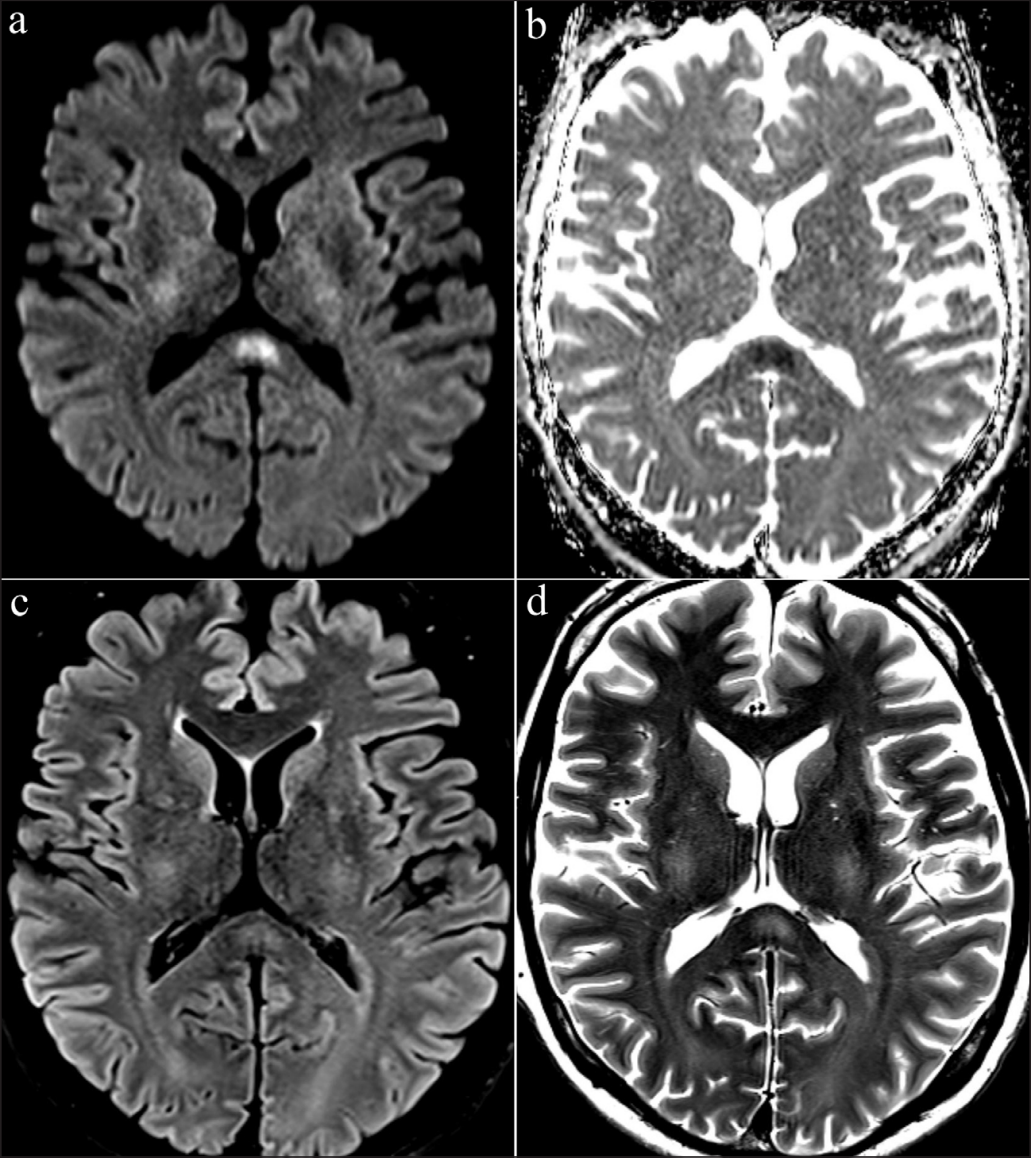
Axial diffusion-weighted image (b = 1000 s/mm^2^) (a) showed a high signal intensity in the splenium of corpus callosum. Apparent diffusion coefficent (ADC) map image (b) showed a low ADC. Axial FLAIR (c) and T2-weighted images (d) showed a slight hyperintense signal at the same location.
